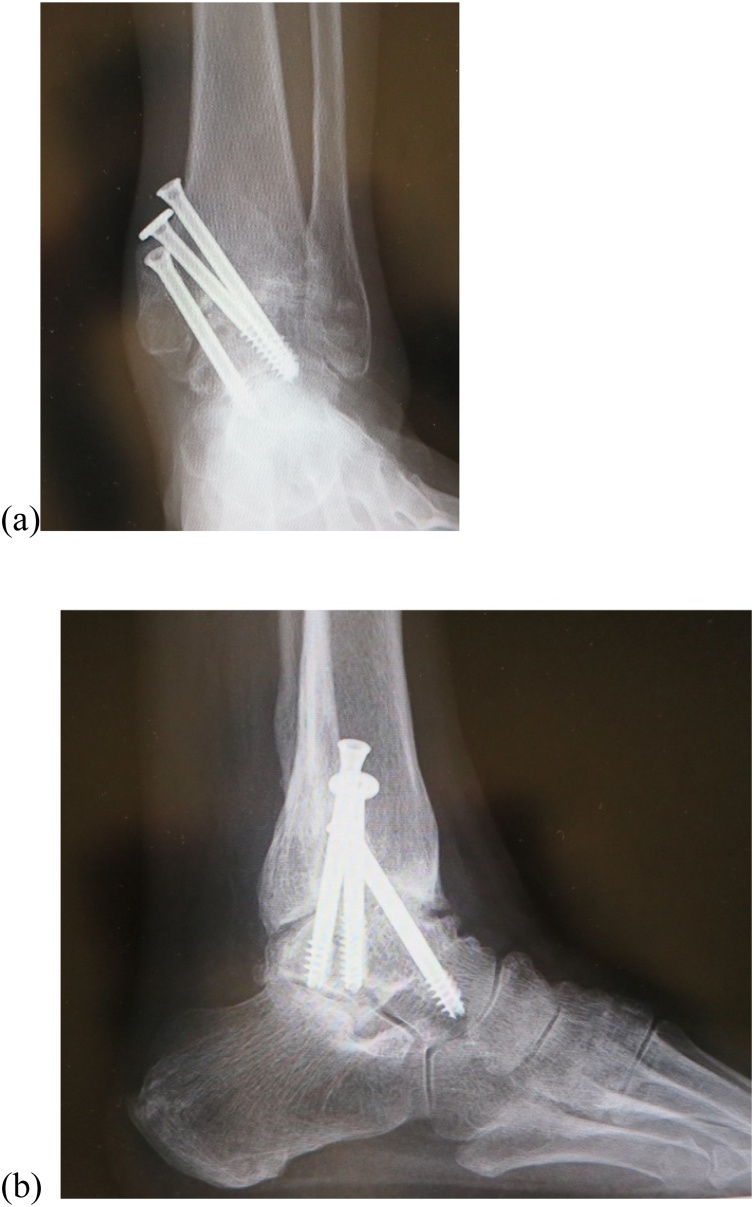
Radiographs 2 years after surgery. Bony union was achieved, seen in the standing position on (a) anteroposterior view and (b) lateral view.
